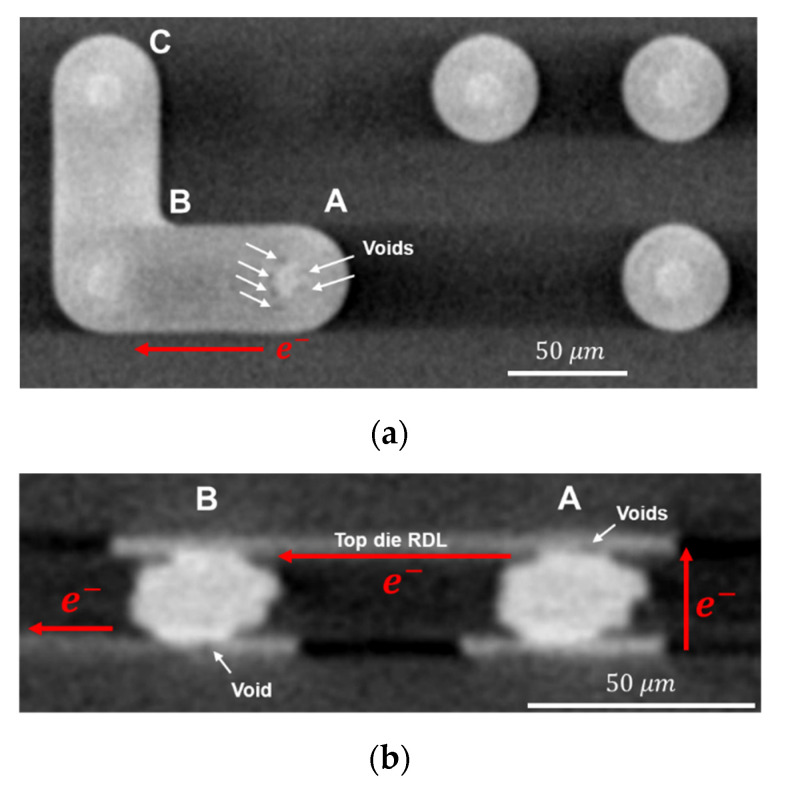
Nondestructive observation of 90 MPa/30 s_K3 bumps. (a) Computed tomography of the top die RDL. The voids form at the top die RDL of Kelvin bump A. (b) Computed tomography of the cross-section along the current direction in Kelvin bumps. The voids are observed at the top die RDL of Kelvin bump A and at the bottom die RDL of Kelvin bump B.
